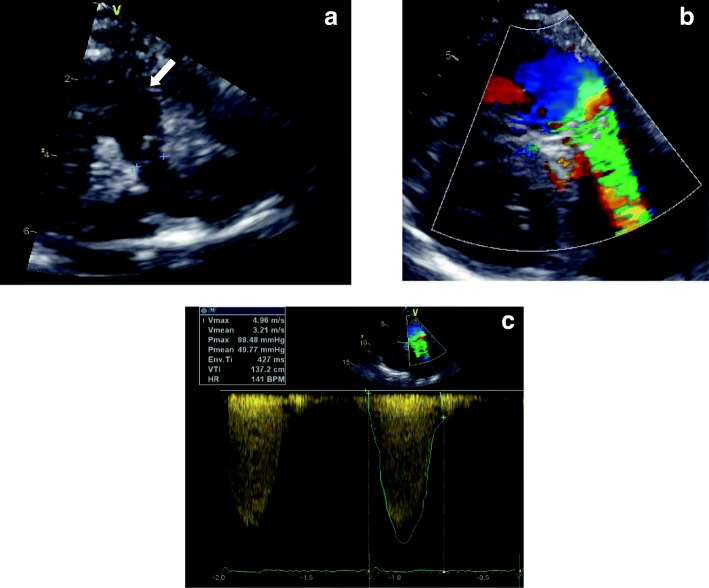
Echocardiography in postoperative TOF. a Parasternal short axis view of pulmonary valve (*crosses*), right ventricular outflow tract (*arrow*), main and branch pulmonary arteries. b Turbulence along stenotic pulmonary valve of an RV to pulmonary artery conduit without stenosis in the RV outflow tract. c Doppler tracing along the stenotic conduit indicates severe stenosis with maximal pressure gradient 98 mmHg
